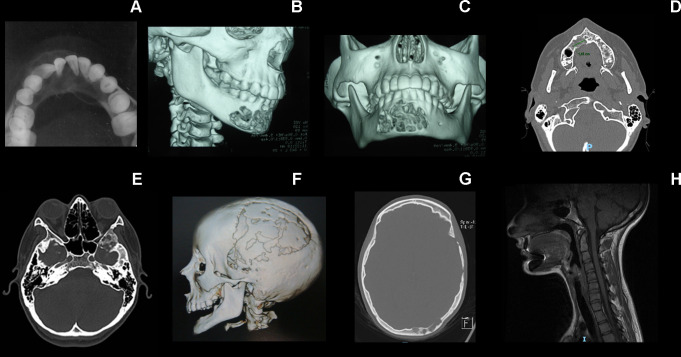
Radiological features of individual 2’s skeletal alterations. (A) Multilocular radiolucent tumour in the anterior part of the mandible causing teeth displacement. (B, C) CT image with 3-D reconstruction showing cortical bone destruction. (D) Axial CT scan showing the primary tumour in the maxilla. (E–G) Osteolytic lesions in the squamous part of the temporal bone, greater wing of the sphenoid, lateral wall of the orbit and diploe of the left occipital bone. (H) Hypoplasia of the vertebral bodies and the intervertebral disc of C2–C3, with fusion of its posterior elements characterising vertebra in block.
